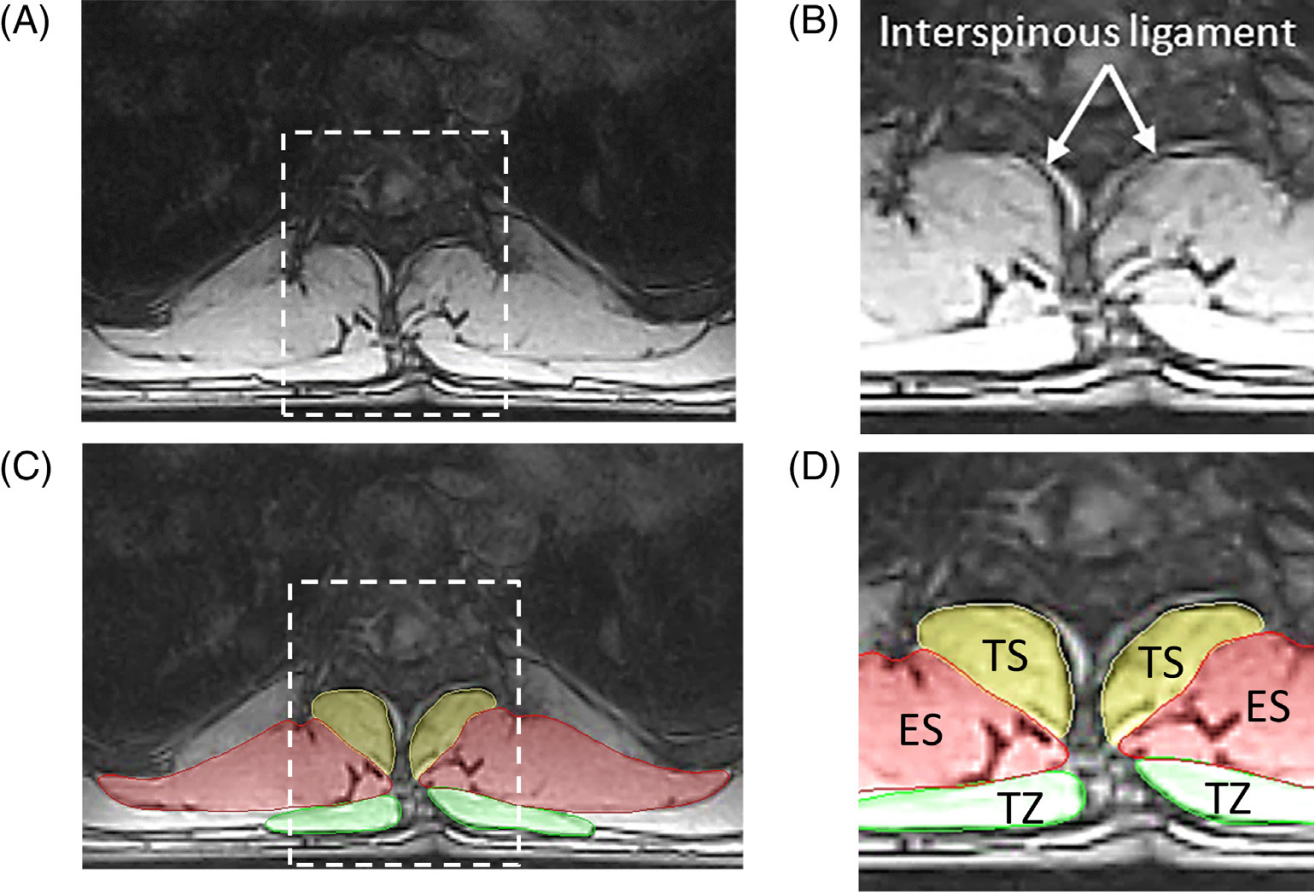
A, Transverse MR image at level T9. B, Zoomed region showing interspinous ligament. C, Transverse MR image at level T9 showing segmented ROIs. D, Zoomed region showing medial border of TS following the interspinous ligament instead of edge of the spinous process. ES, erector spinae; TS, transversospinalis; TZ, trapezius
